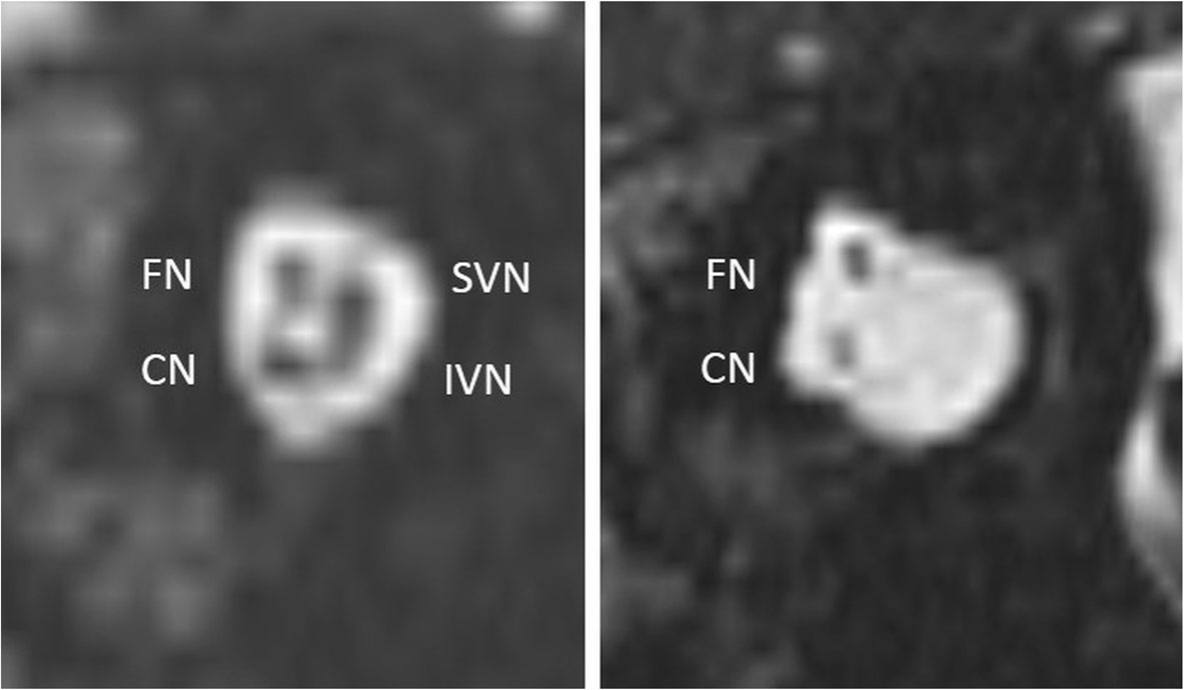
A 57-year-old female patient with unilateral SNHL from birth. MRI 3D-CISS parasagittal image of the internal auditory canal shows regular facial nerve (FN), cochlear nerve (CN), superior vestibular nerve (SVN), and inferior vestibular nerve on the healthy side (left image) and missing SVN and IVN, as well as a hypoplastic CN on the diseased side (right image)
